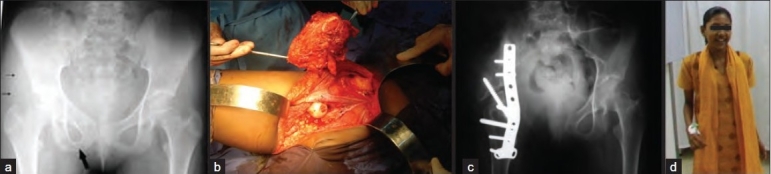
(a) Preoperative X-ray (anteroposterior view) of a patient showing Ewing’s sarcoma involving right ischium (thick black arrow) and soft tissue extent (thin black arrows). (b) Intraoperative clinical picture of same patient showing Type I+II pelvic resection. (c) Postoperative X-ray (anteroposterior view) after Type I + II resection and attempted iliofemoral fusion with plate osteosynthesis after 3 years. (d) Clinical followup after 3 years with good functional outcome
